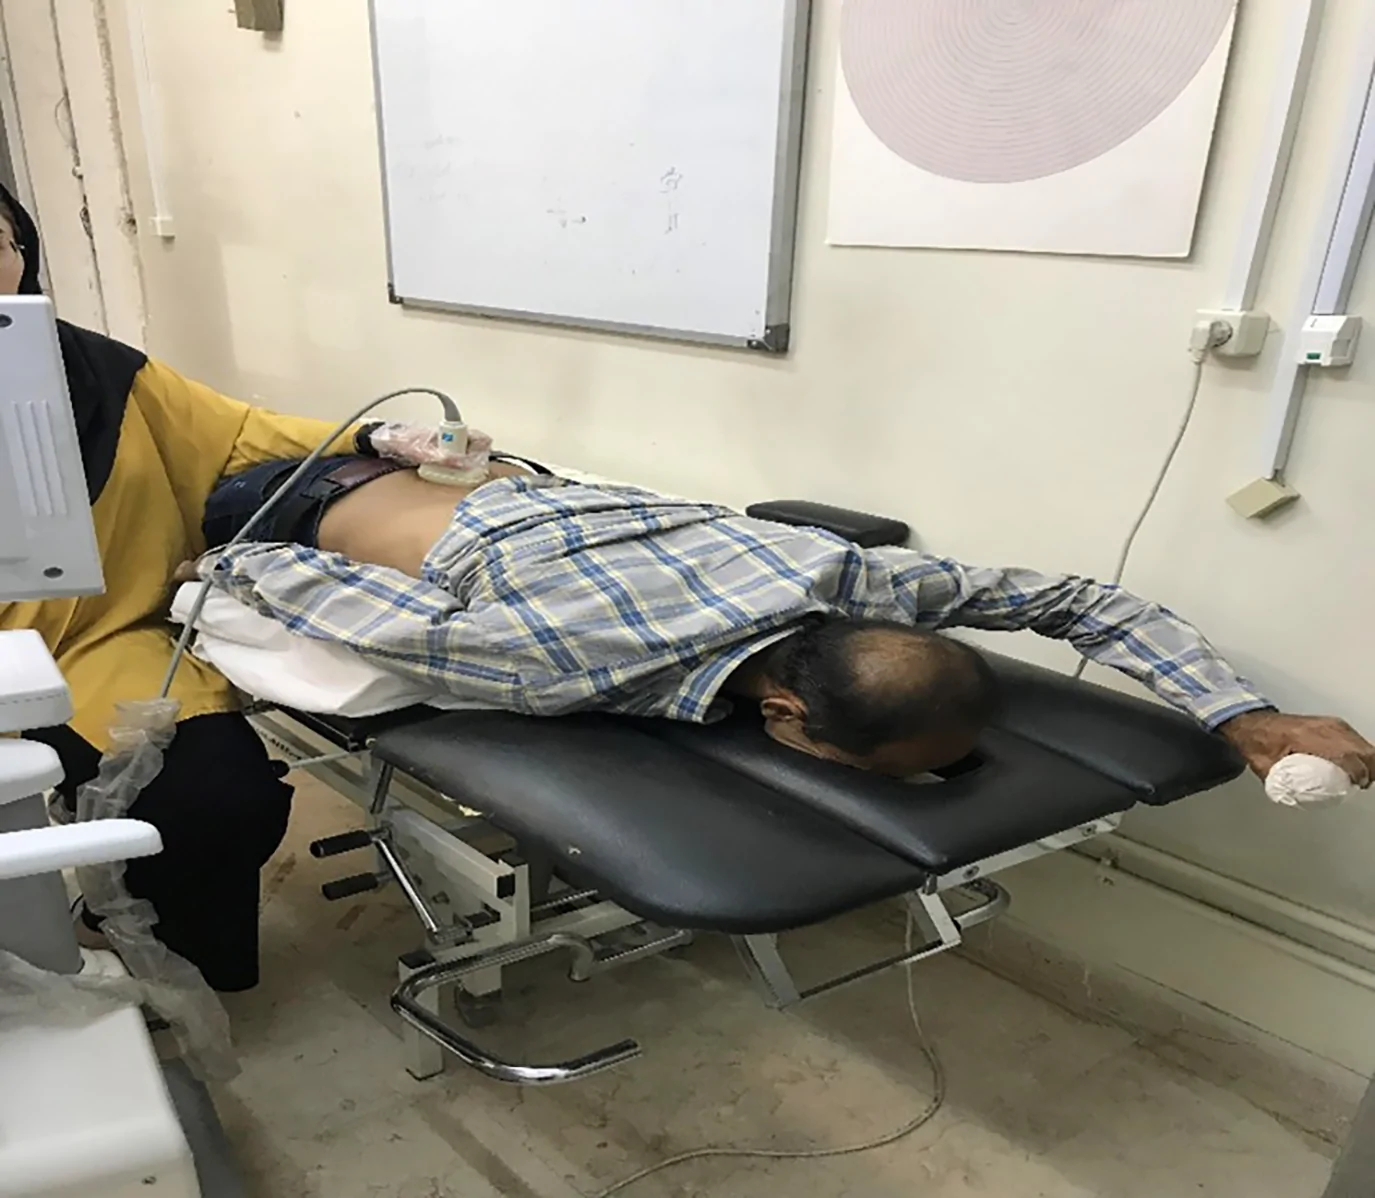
Ultrasound image showing of the thickness of the Multifidus muscle in the prone position during contraction. Created by the authors. Published under CC BY 4.0.
